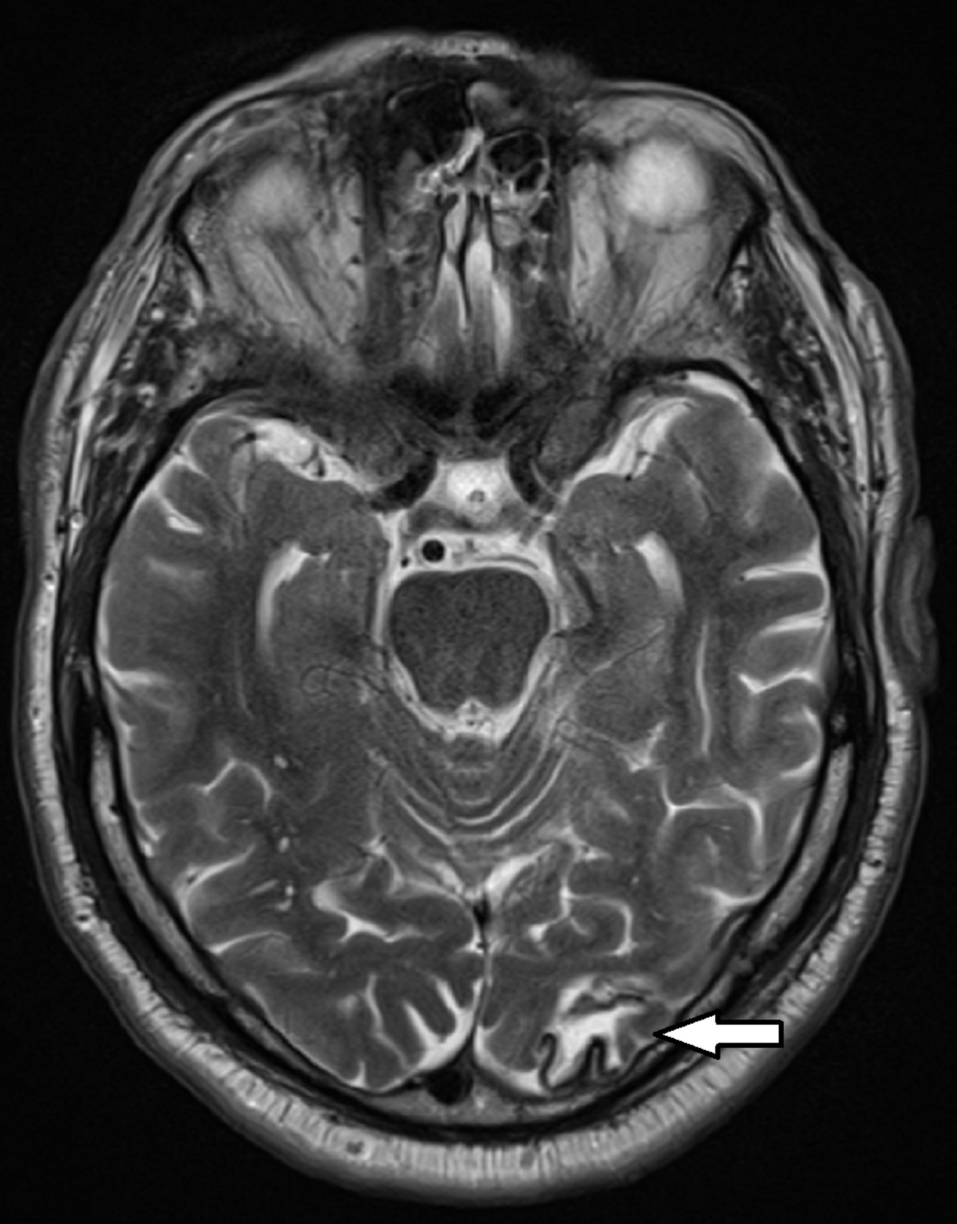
Patient’s magnetic resonance imaging showing no acute infarct and encephalomalacia of the left occipital lobe (arrow), consistent with the chronic appearance of prior infarct.
